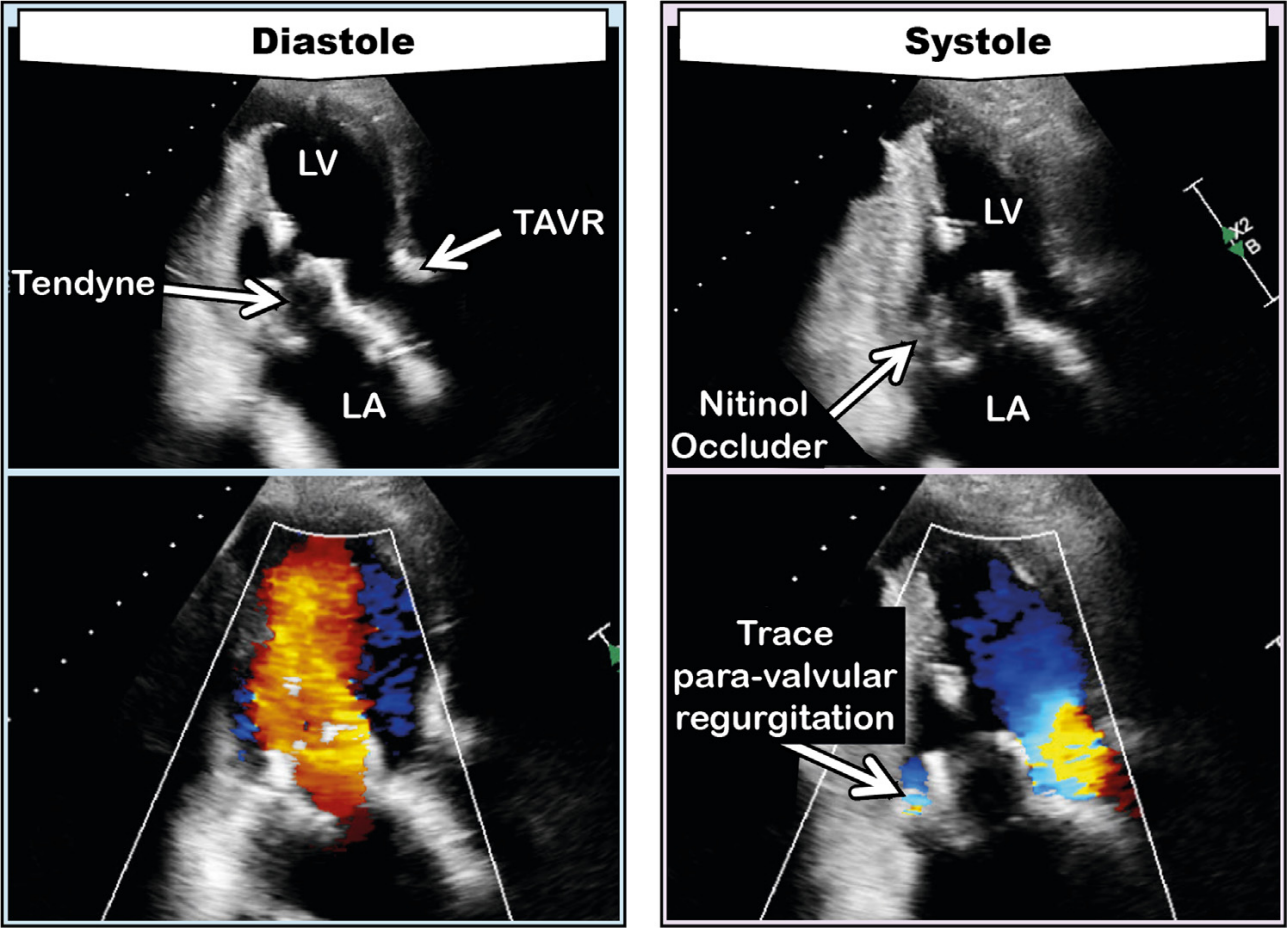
Transthoracic Echocardiogram 3 Months Postprocedure Showing Trace Paravalvular Mitral Regurgitation Abbreviations as in Figure 1 and the Visual Summary.
